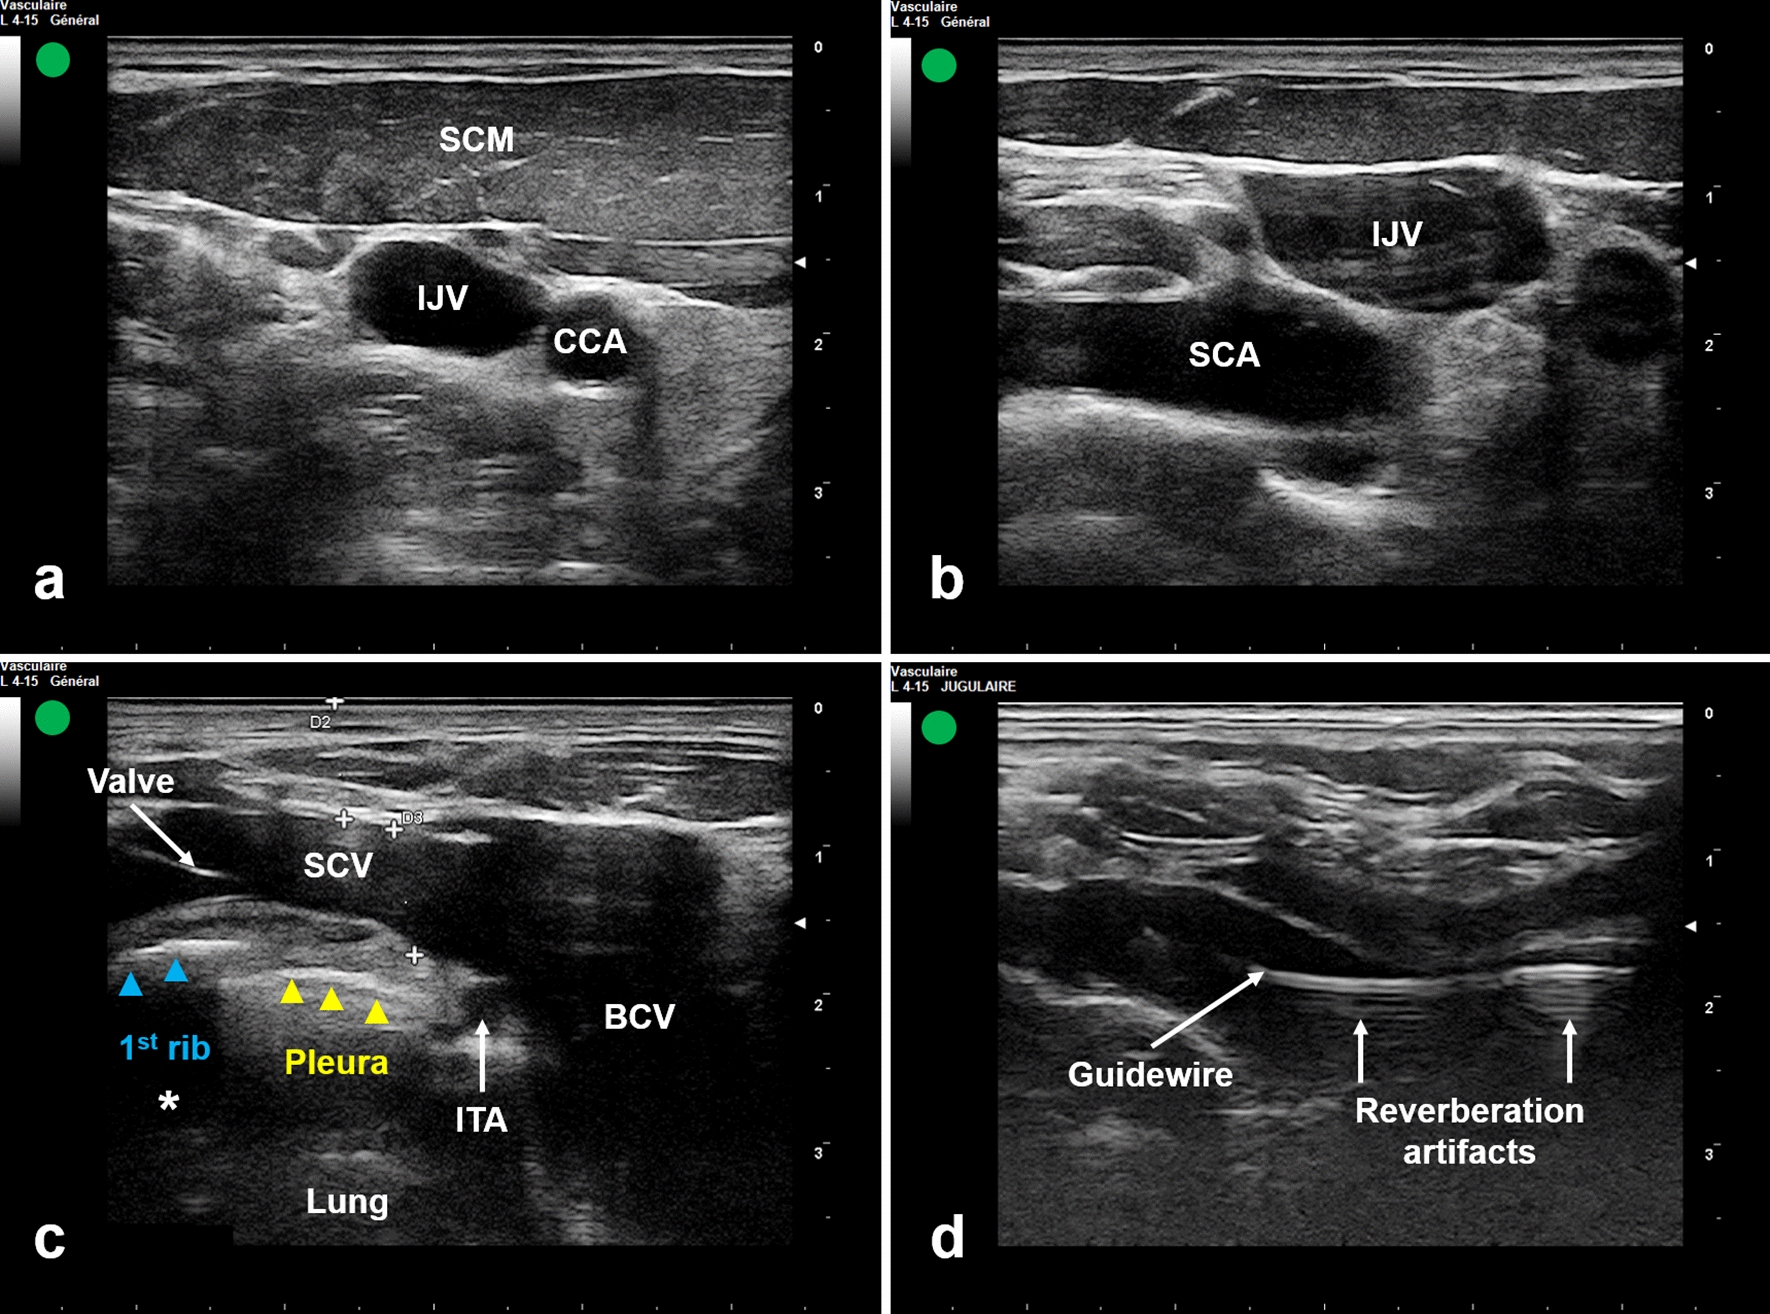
Ultrasound-guided subclavian vein catheterization using the long-axis view of the vein via the supraclavicular approach in combination with in-plane needle approach. a Ultrasound short-axis view of IJV. b Ultrasound identification of IJV and SCA in the supraclavicular fossa. c Ultrasound long-axis view of SCV and BCV. d Ultrasound visualization of the guidewire in the SCV. *SCV* subclavian vein, *SCA* subclavian artery, *BCV* brachiocephalic vein, *ITA* internal thoracic artery, *Asterisk* acoustic shadow of the 1st rib
